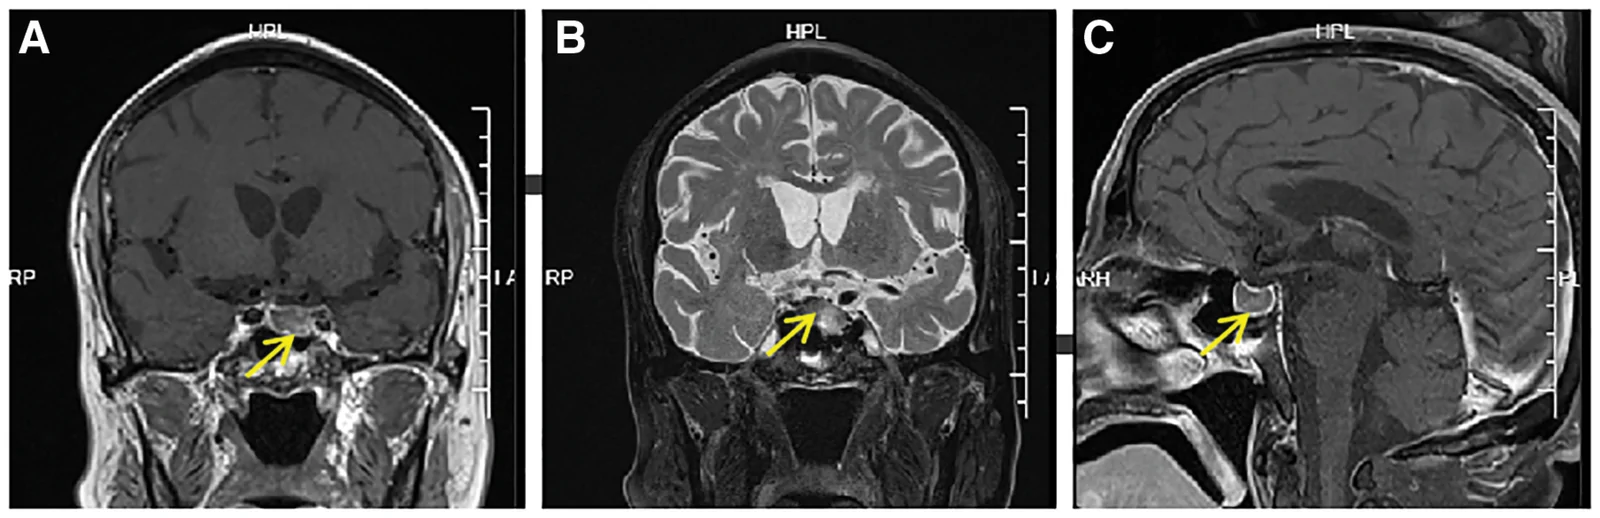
Enhanced MRI of the pituitary gland. (A) Coronal T1-weighted images, (B) Coronal T2-weighted images demonstrated that the pituitary stalk was not clearly displayed with mixed abnormal signal. (C) Sagittal T1-weighted images showed rim enhancement after gadolinium injection (highlighted by the arrow).
